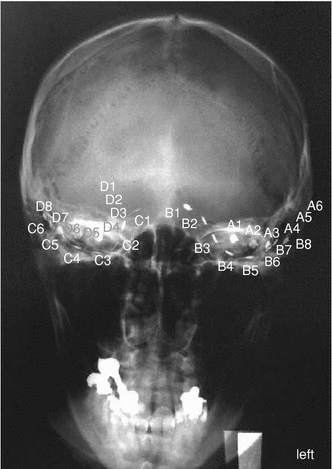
X-ray image of patient A in *frontal view* indicating electrode positions of the four strip electrodes: *A* and *B* below the left temporal lobe, *C* and *D* below the right temporal lobe
